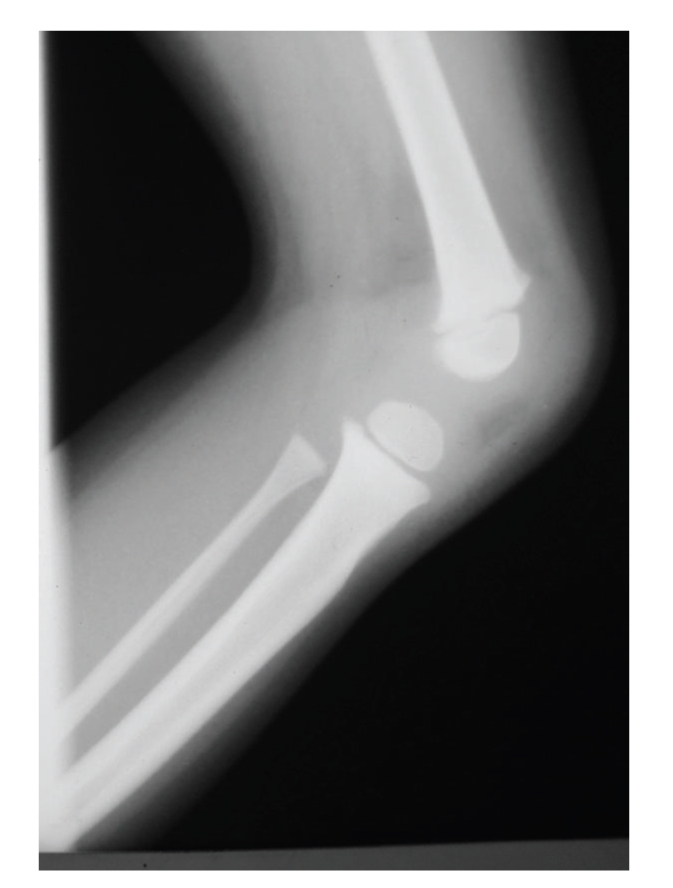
Knee lateral radiographs revealed absence of the patella.
